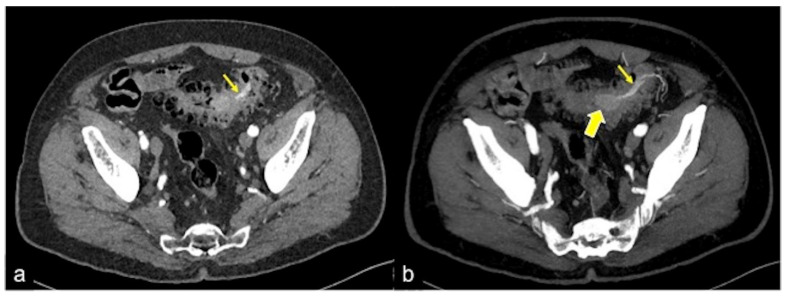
CTA multiphasic study of pedunculated polyp of the sigma. In the arterial phase (a) and relative MIP (b), it is possible to define an arterial vascular axis (a,b, short arrow) supporting a large polyploid formation (b, large arrow).
